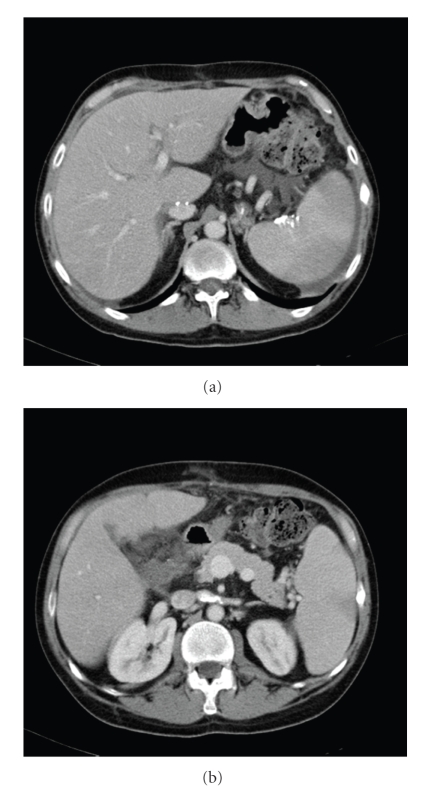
Thoracoabdominal computed tomography: (a) multiple splenic infarctions, (b) radio-opaque material in the left renal vein and the splenic hilum corresponding to cyanoacrylate emboli.
